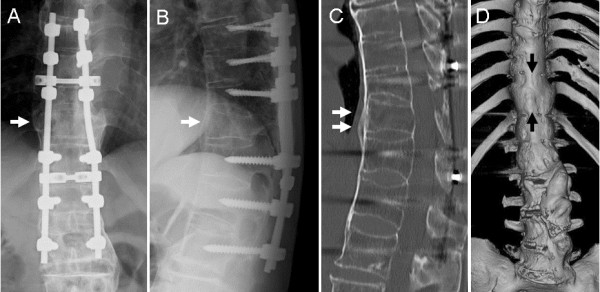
Anteroposterior (A) and lateral (B) radiographs taken eighteen months after surgery of a patient with Parkinson’s disease with a thoracic spine fracture in a severely ankylosed spine show bony union of the fracture site (T11: arrows) is achieved (arrows) with no evidence of instrument failure. Sagittal (C) and three-dimensional (D) images of computed tomography on the same day show more clearly the complete bony fusion (arrows).
